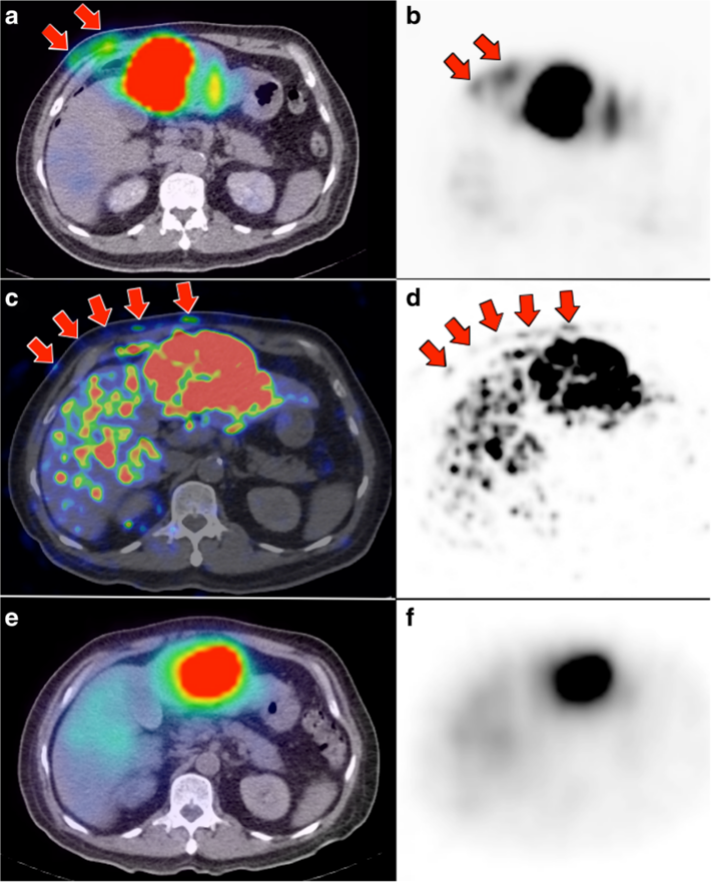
Patient 22.^90 ^Y radioembolization of part of a segment III HCC via the terminal branch of the right internal mammary artery (RIMA). (a, b) Pre-therapy ^99m^Tc MAA SPECT/CT demonstrates non-target activity in the right lower anterior chest wall (arrows), shunted from proximal branches of the RIMA. (c, d)^90^Y PET/CT depicts in high-resolution, non-target activity conforming to the anatomy of the right lower anterior chest wall, concordant with ^99m^Tc MAA SPECT/CT. The slight dissimilarity in chest wall activity biodistribution between ^90^Y PET/CT versus ^99m^Tc MAA SPECT/CT is due to local arterial flow changes after prophylactic bland embolization of proximal branches of the RIMA (images not shown). (e, f)^90^Y bremsstrahlung SPECT/CT was unable to detect any non-target activity in the chest wall due to its low image resolution.
